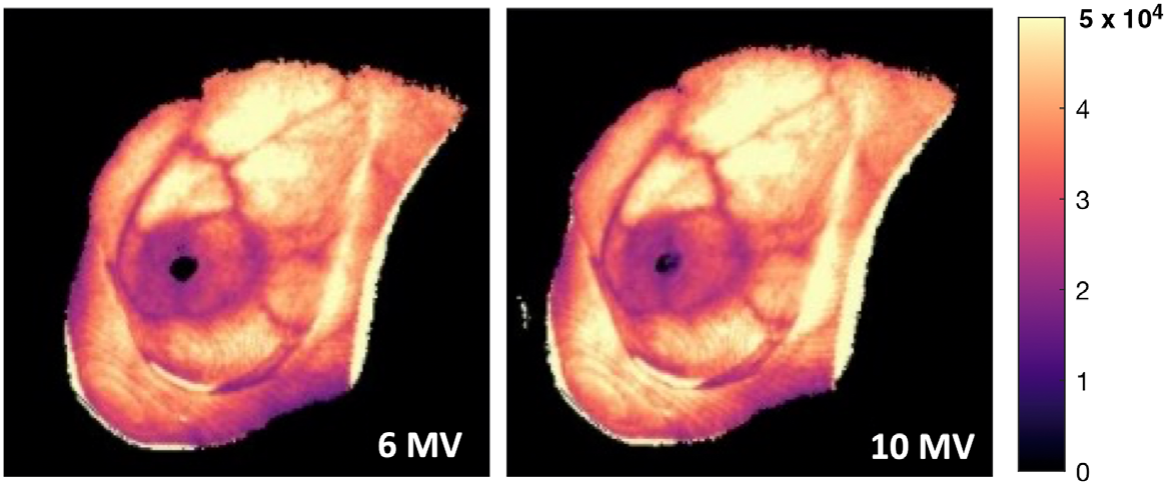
Comparison of cumulative Cherenkov images taken during whole-breast radiation therapy at 6 versus 10 MV x-rays for a single patient.^6^ The observed Cherenkov intensity remains approximately constant, even at higher x-ray energies.
